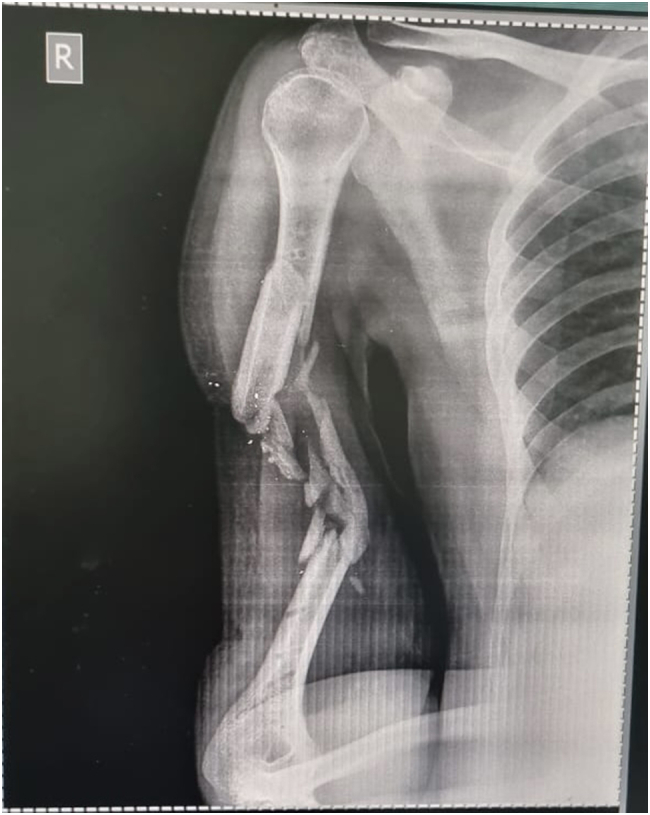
X-ray of the right humerus anteroposterior (AP) view after removal of external fixator before final fixation.
